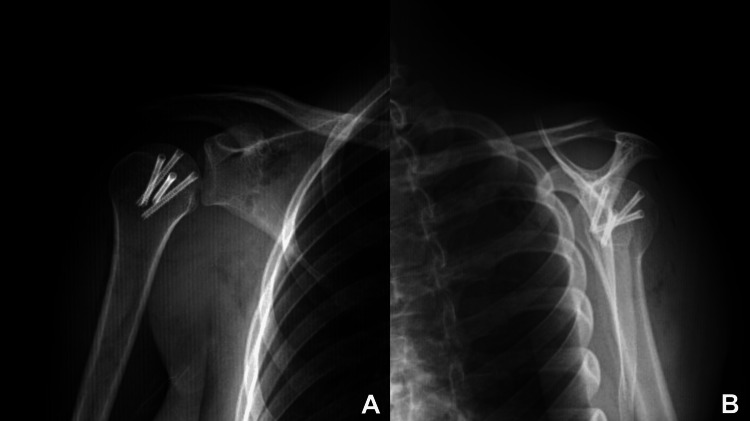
Immediate postoperative anteroposterior (A) and axial (B) radiographs
